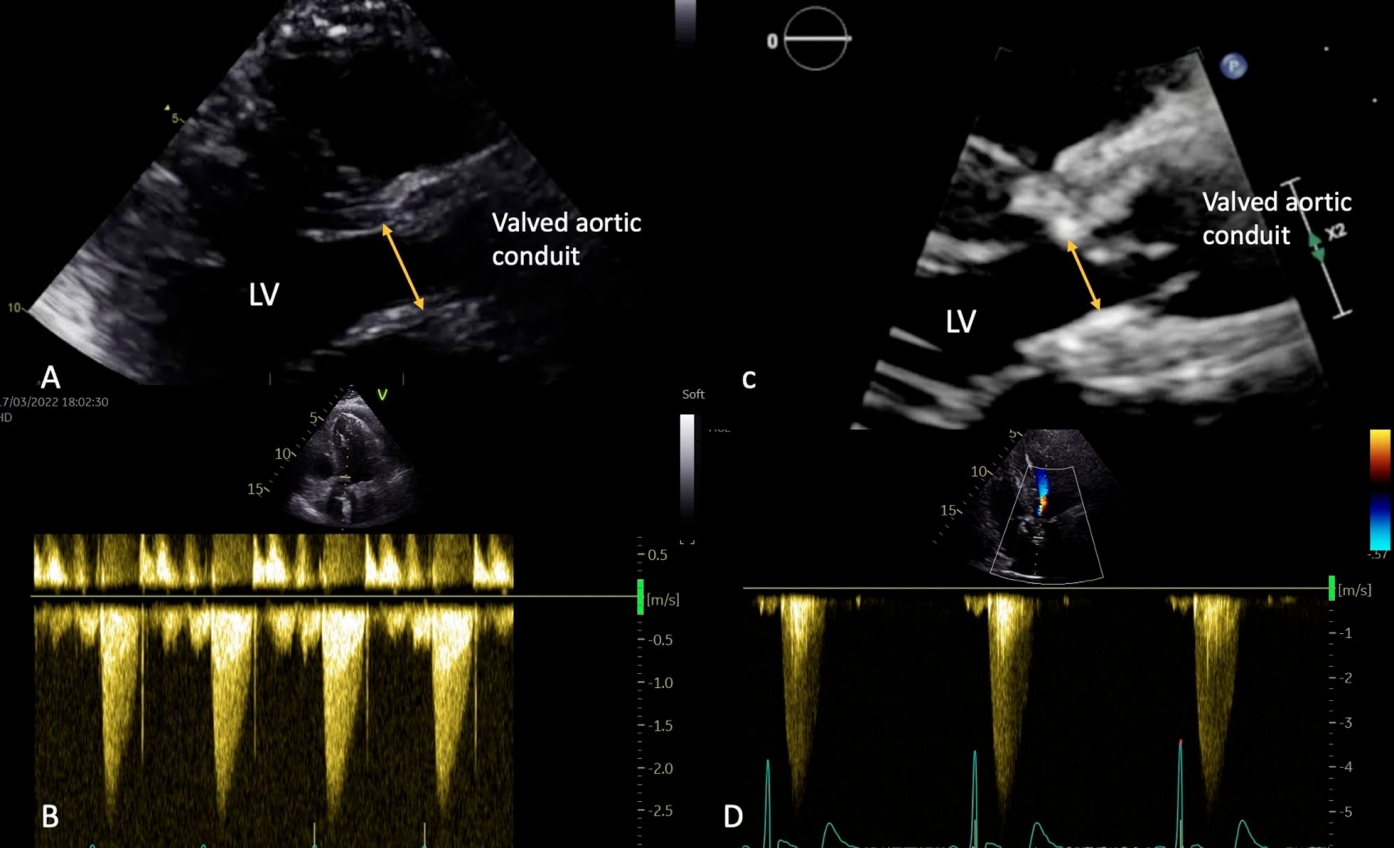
Transthoracic echocardiography, long axis parasternal view with same patient early post-operatory (A) and (C) and 1-year follow up exam (B) and (D), showing a shrinkage of the aortic annulus at 1 year (measured at the level of the yellow arrows), from 18 to 14 mm (A) and (C) together with a transaortic mean gradient and maximal velocity by Doppler continuous wave interrogation, from 2,4 m/sec and 12 mmHg (B) to 4,6 m/sec and 59 mmHg (D)
